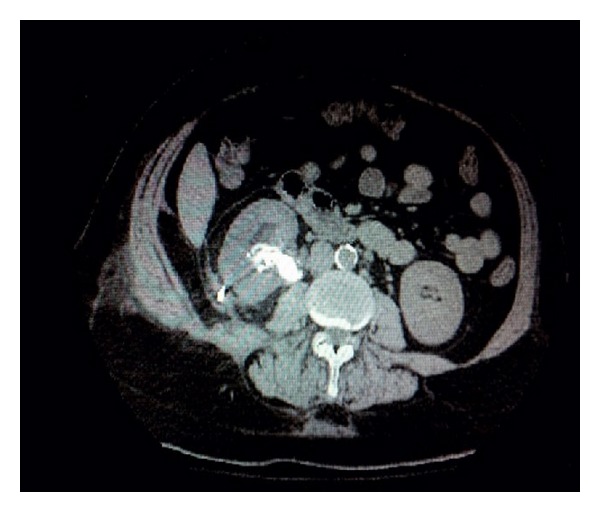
CT scan of the abdomen and pelvis showing a right staghorn calculus, right hydronephrosis with air in the collecting system, and perinephric stranding.
